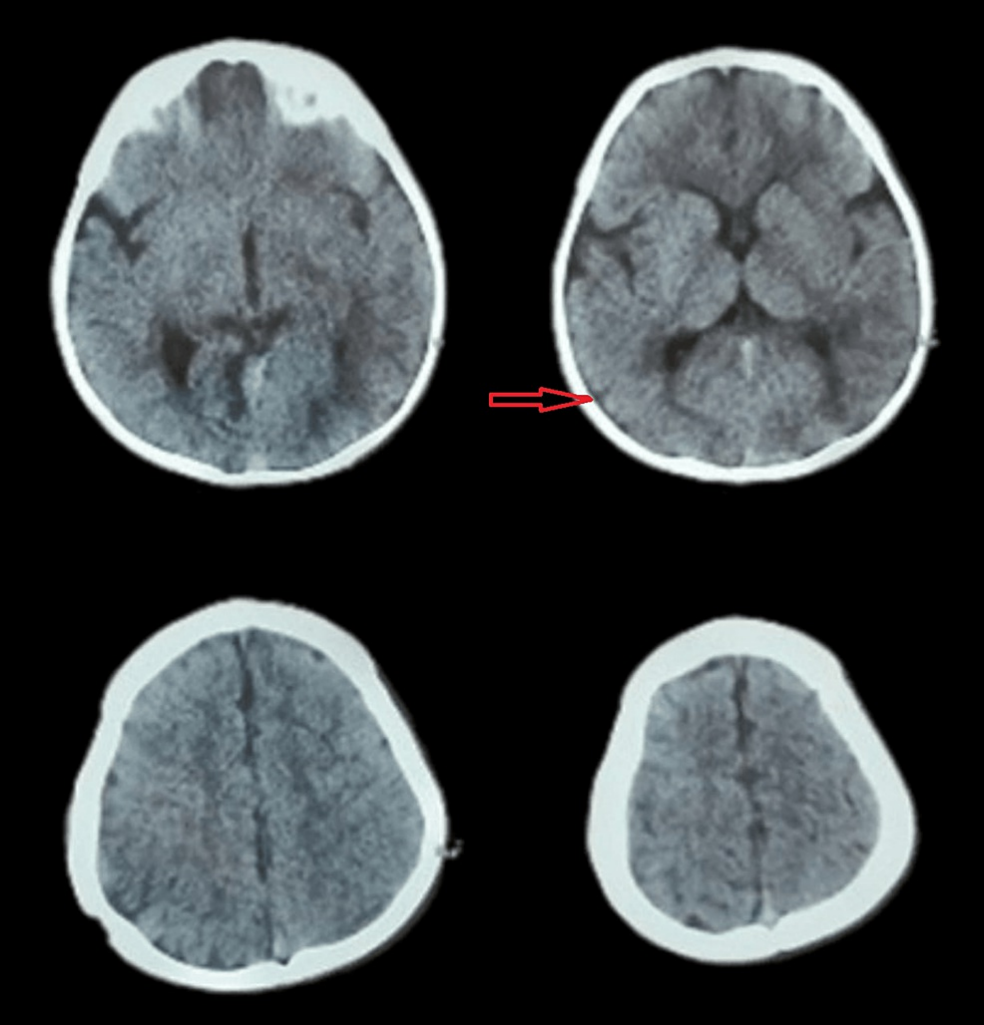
Radiological imaging post-surgery Orange arrow: CT brain showing the resolution of subdural hematoma CT: computed tomography
